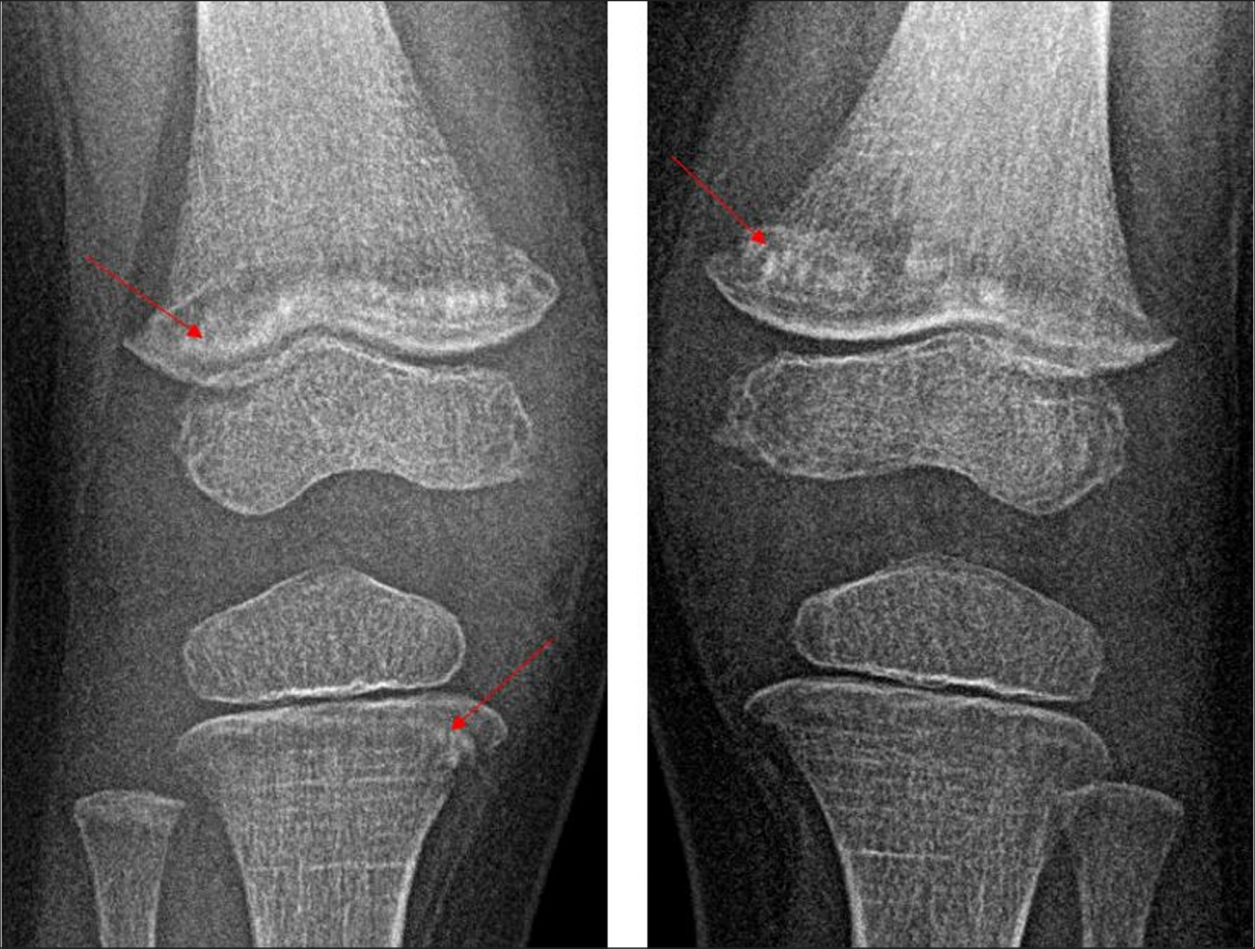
Further investigation with magnetic resonance imaging (*Figure 3*) was done and revealed high T2-signal at the level of the metaphyseal bands (arrow) with adjacent soft tissue oedema (*Figure 3*, arrowhead). The pathological fractures were also visible as T1 hypo-intense lines (dotted arrow).
